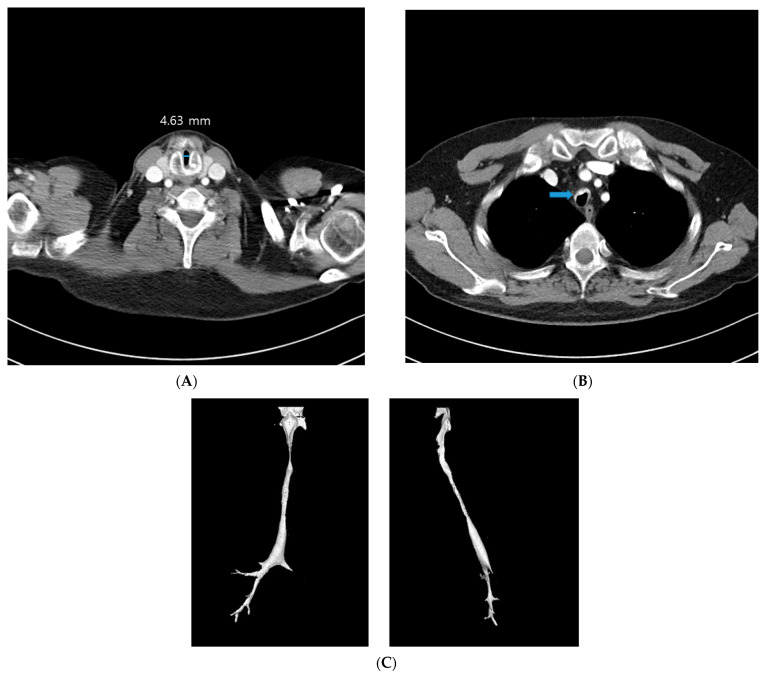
(A) CT shows subglottic stenosis at cricoid level with short-axis diameter 4.63 mm. (B) Calcification and anterolateral wall thickening of tracheobronchial tree (arrow) and resulting luminal narrowing. (C) Tracheobronchial tree shows irregular narrowing of the lumen and marked diffuse luminal-narrowing of left mainstem bronchus.
